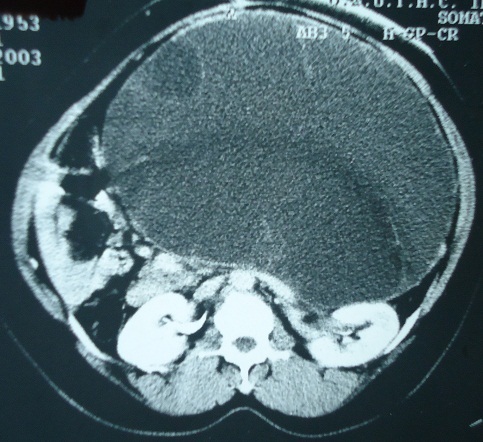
Huge mucinous cystadenoma of the pancreas CT Abdome
